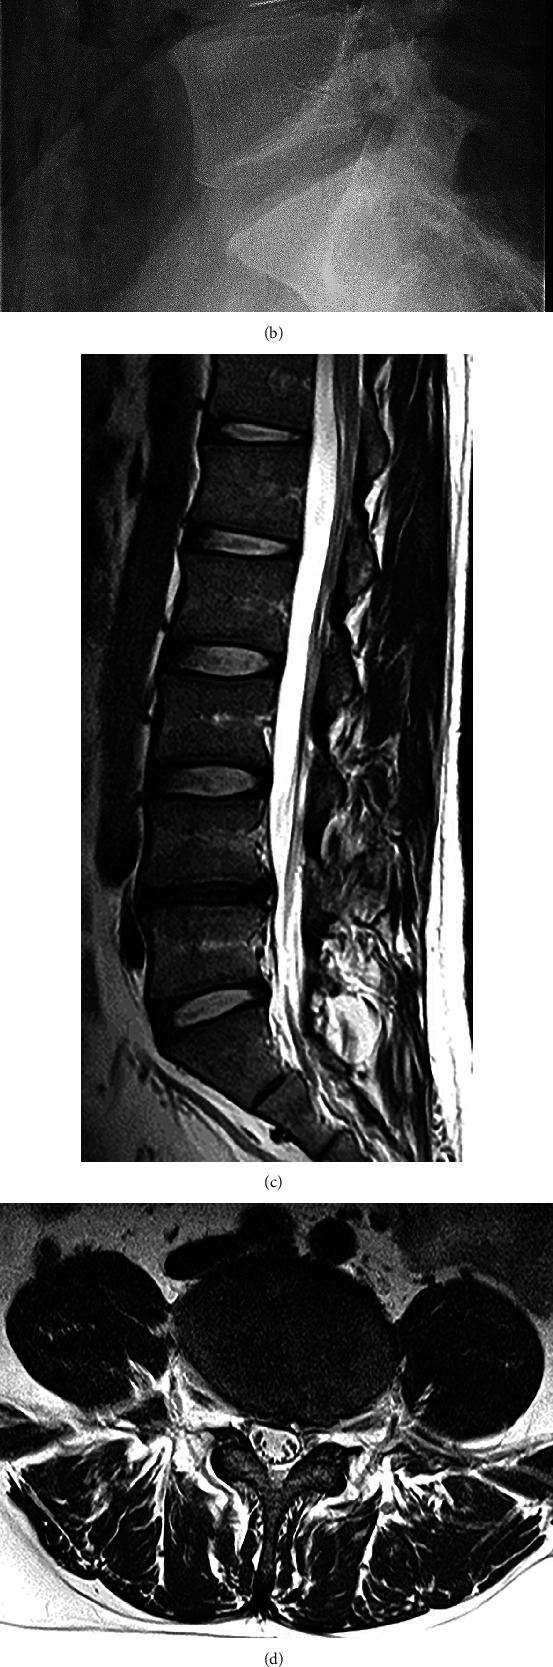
Condoliase injection procedure and findings after treatment. In the prone position, a 12 cm spinal needle was inserted from the left lateral side under fluoroscopy, advanced to the center of the L4/5 disc (a, b), and the condoliase was injected. Two months after treatment, lumbar T2-weighted magnetic resonance imaging showed that the disc herniation at L4/5 had significantly decreased in size (c, d). Although progression of the degree of affected-disc degeneration was observed (c), standing radiography revealed that the L4/5 disc height was the same as that before treatment (e).
